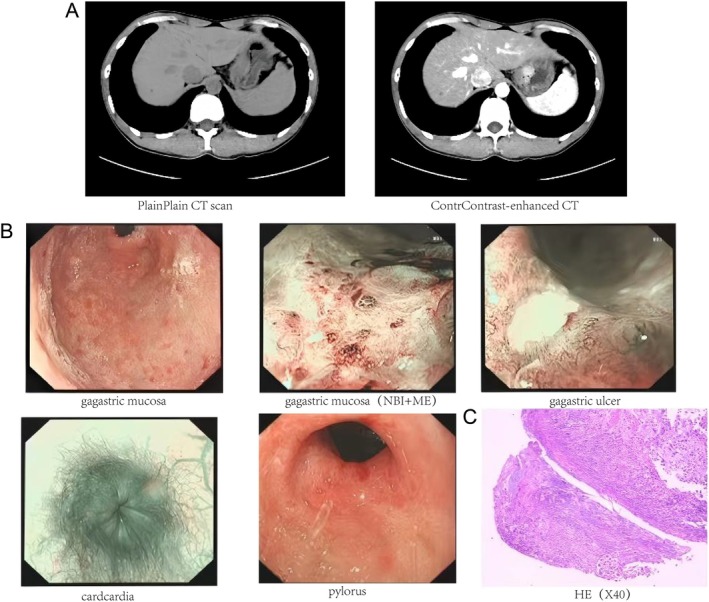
Abdominal Contrast‐Enhanced CT, Second Endoscopic and Pathological Examination (January 22, 2025). (A) There is pronounced thickening of the gastric wall near the cardia. The enhanced scan reveals multiple small lymph nodes within the perigastric region. (B) The gastric mucosa exhibits total atrophy, with multiple scar‐like alterations. A substantial depressed ulcer is observed on the anterior wall at the junction of the antrum and corpus. (C) Pathological examination reveals ulcer formation with atypical cells present within necrotic tissue, alongside mild atypical hyperplasia in certain glands.
